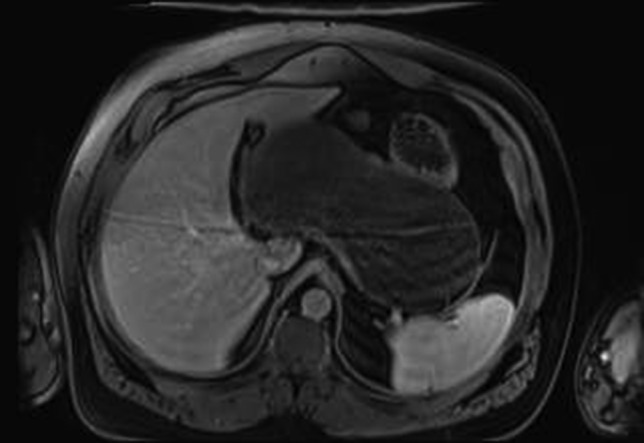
MRI imaging showing a cystic mass located between gastric antrum, pancreas, spleen and hepatic hilum.
